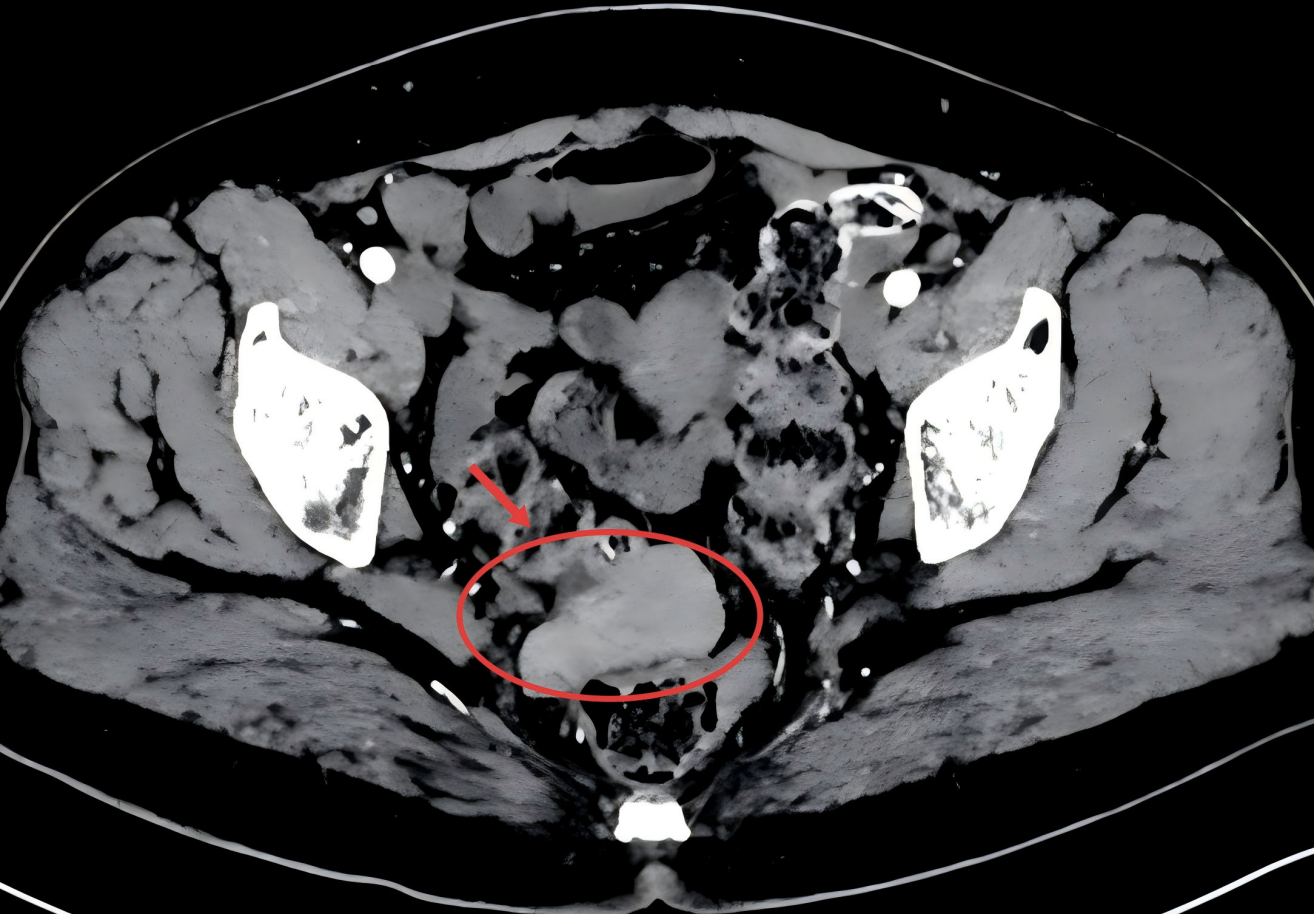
Contrast-enhanced CT of the abdomen. Red arrows indicate the nodular lesions in the rectouterine pouch.
